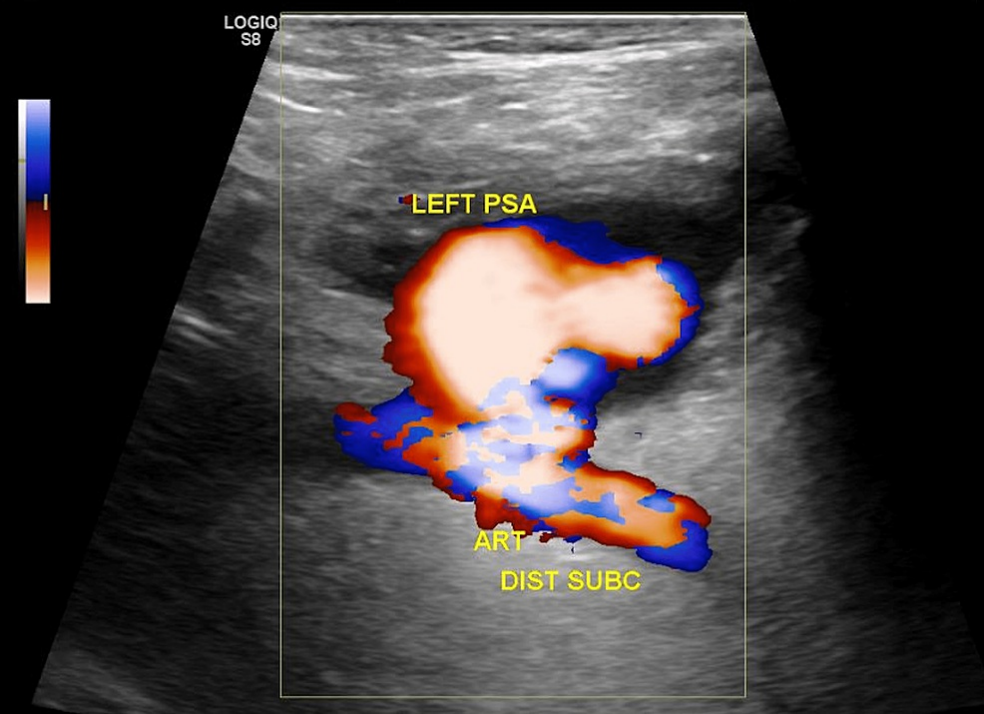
Arterial duplex ultrasound of the left upper extremity showing a distal subclavian artery PSA measuring 3.8 x 6.5 cm with a neck base of 1.2 cm. PSA: pseudoaneurysm.
